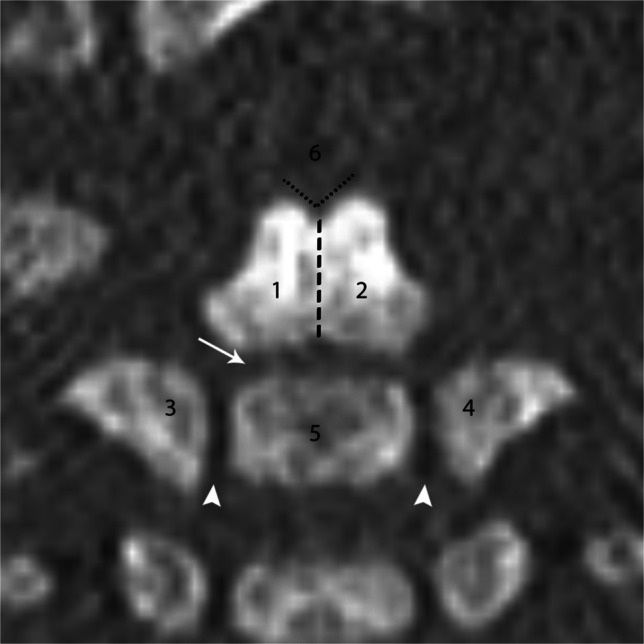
Coronal CT of the C2 vertebral body in a 1-month-old male. C2 has six ossification centers. Two make up the dens (*1* and *2*), two neural arches (*3* and *4*), the centrum (*5*), and the tip of the dens (*6*), which is not yet ossified. There are six synchondroses. The mid-dens synchondrosis (*dashed line*), posterior neural arch (not visualized), subdental (*arrow*), two neurocentral (*arrowheads*), and apicodental (*dotted*
